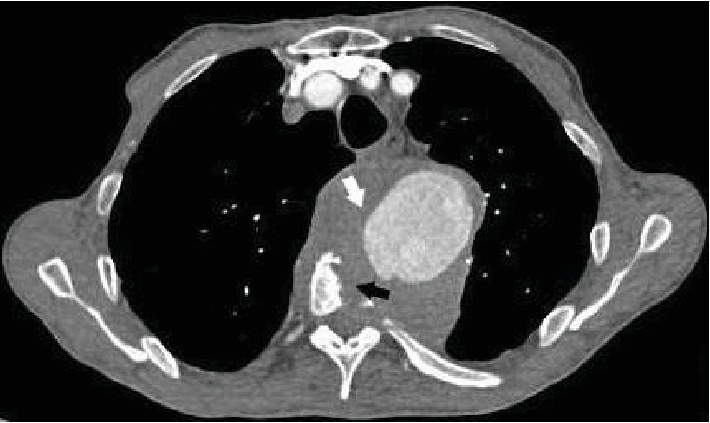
Axial section of a chest CT angiography showing aneurysmal formation (white arrow) implanted between the butt and the descending aorta, partially thrombosed coming into direct contact with the anterior surface of the vertebral bodies of the dorsal spine, responsible for bone lysis (black arrow) with a positive draped aortic sign.
